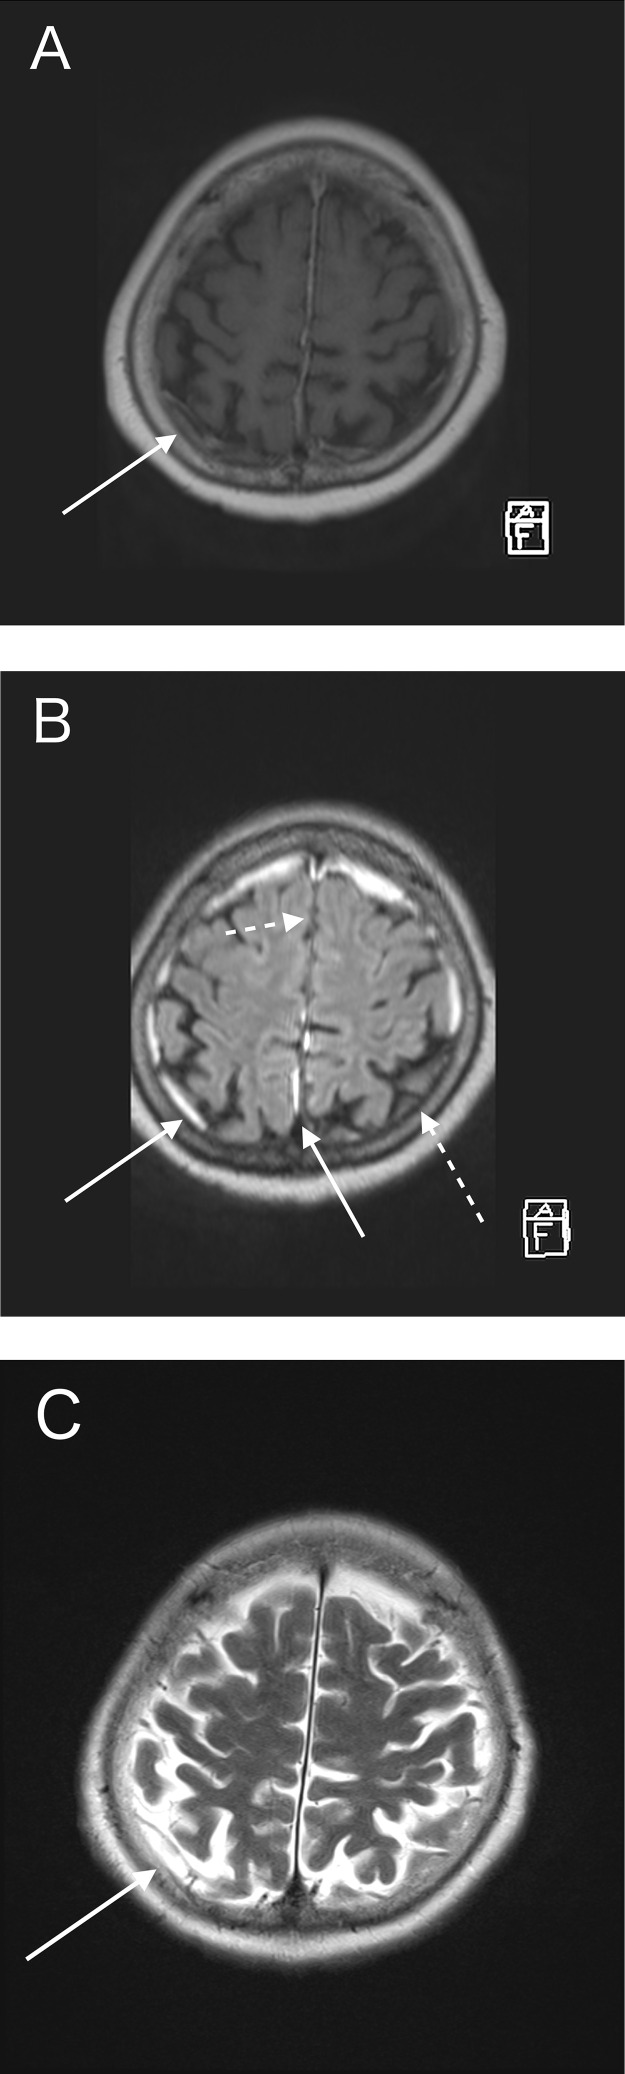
(A) CE-T1WI showed enhancement of the convexity of the dura and falx (arrow). (B) CE-FLAIR prominently visualized these lesions (arrows) against the backdrop of nonenhanced normal dura (dashed arrows). (C) T2WI demonstrated high intensity in the thickened convexity dura mater (arrow).
